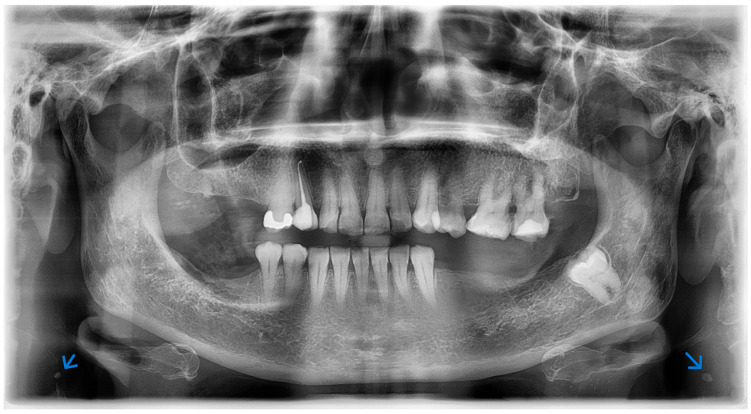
Example of a panoramic dental radiograph showing a calcified carotid atheroma (blue arrow).
